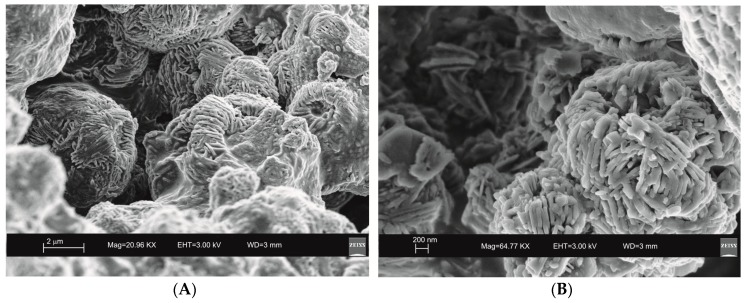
SEM images of sodaline characterizing the sample 6 months after the ultrasonic water bath treatment. (A) overview of zeolites formed; (B) detail of sodalite crystal.
